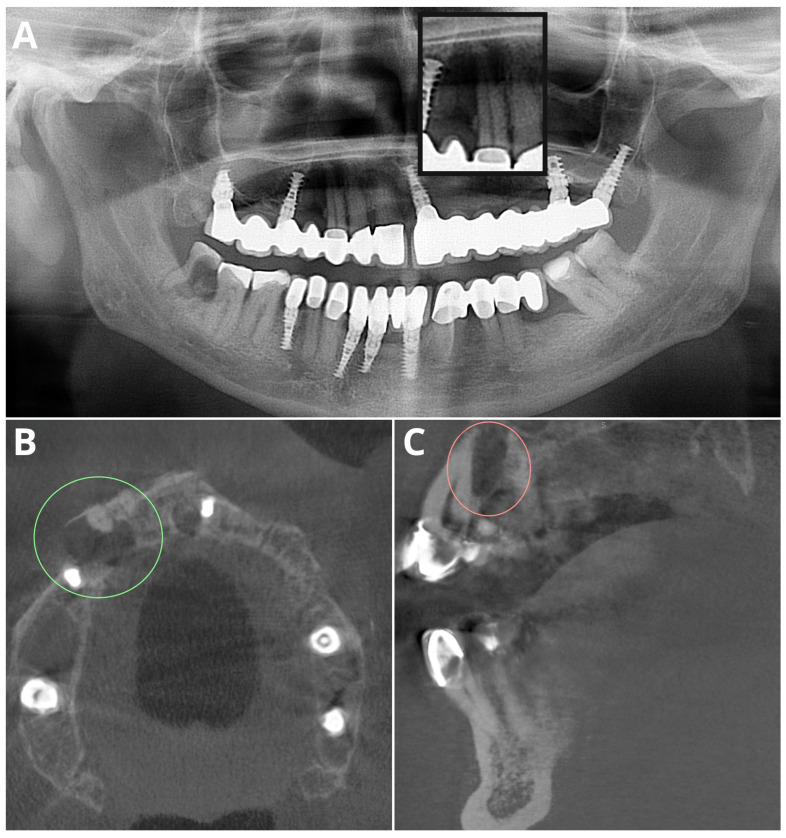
Sample false negative AI diagnosis in both OPG and CBCT evaluations, caused by high image noise from metal artifacts. In the OPG image, the lesion is magnified in the black rectangle (A). CBCT images of PL in color ovals in the axial plane (B) and sagittal plane (C).
